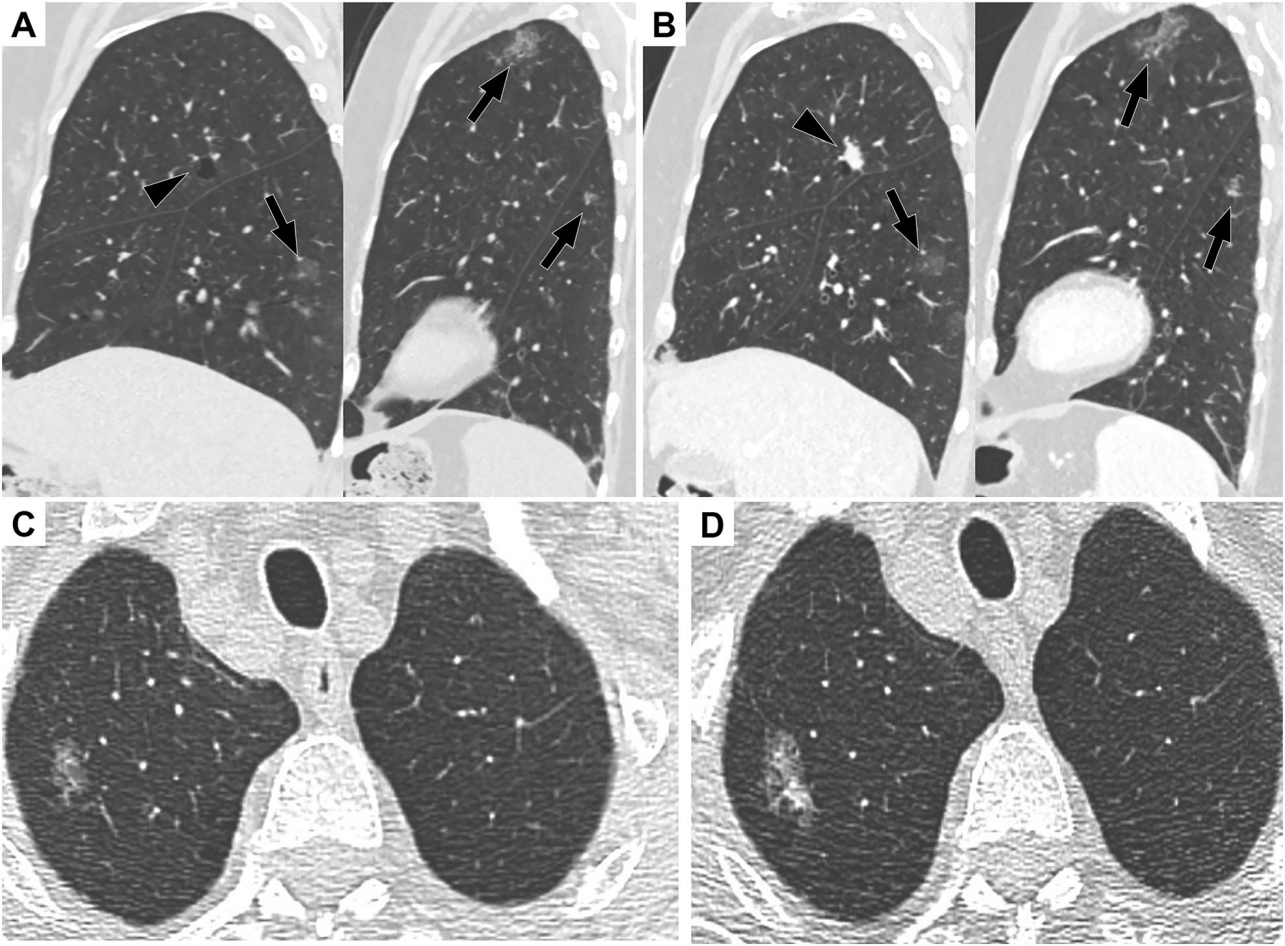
CT follow-up in a patient with subsolid nodules. A Pure ground glass nodules of the right lower lobe and the left upper and lower lobes (arrows). B Four years later (standard dose contrast-enhanced CT), the pure ground glass nodules were unchanged, whereas a spiculated solid nodule corresponding to an invasive adenocarcinoma had developed in the lung cyst wall (arrowhead). C A pure ground glass nodule was found in the apical segment of the right upper lobe. D Three years later, the nodule had grown and developed a solid portion posteriorly
